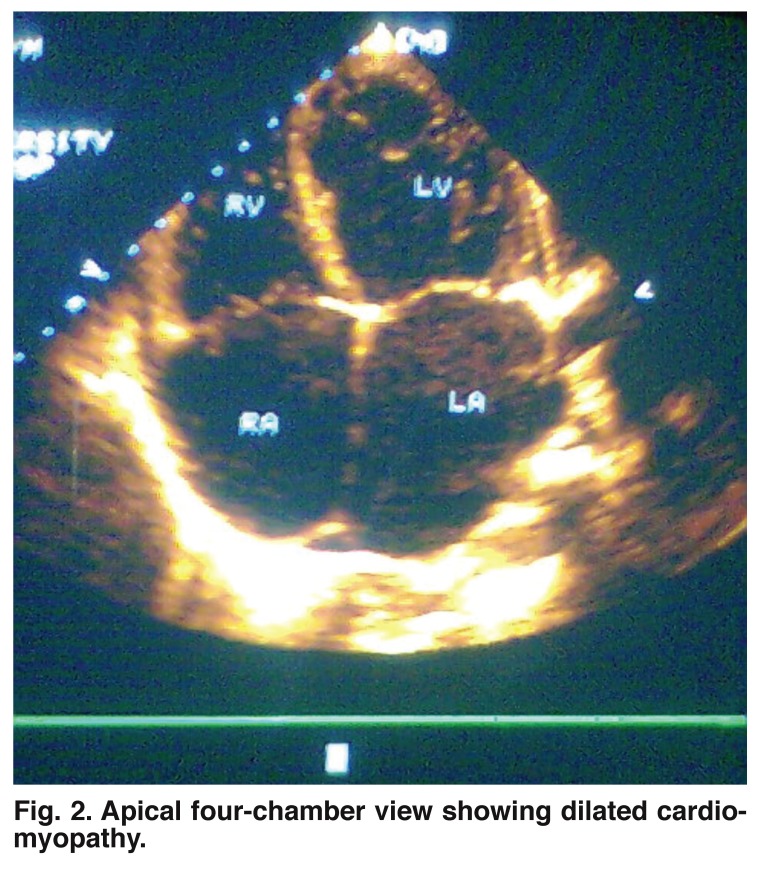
Apical four-chamber view showing dilated cardiomyopathy.
